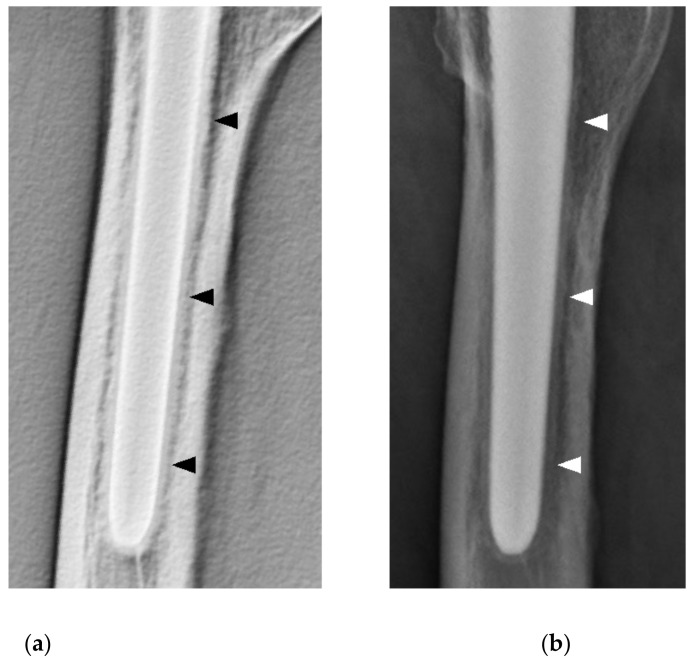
An 83-year-old female patient 3 y after THA. (a) DTS (lateral view); there was bone formation surrounding the stem (black arrowheads). (b) Plain radiographs (lateral view) of the same patient as in (a); there was cancellous condensation similar to a reactive line (white arrowheads). THA, total hip arthroplasty; DTS, digital tomosynthesis.
